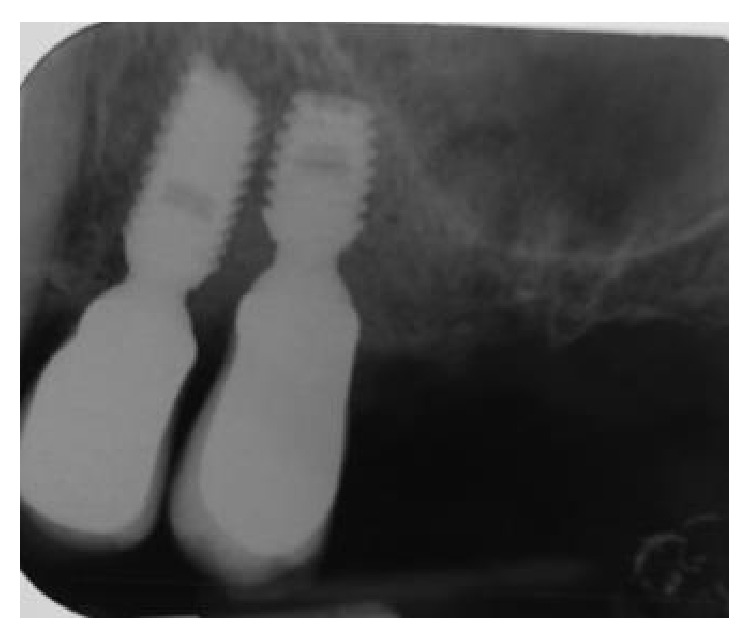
Postoperative radiograph indicating complete filling of the peri-implant defect.
